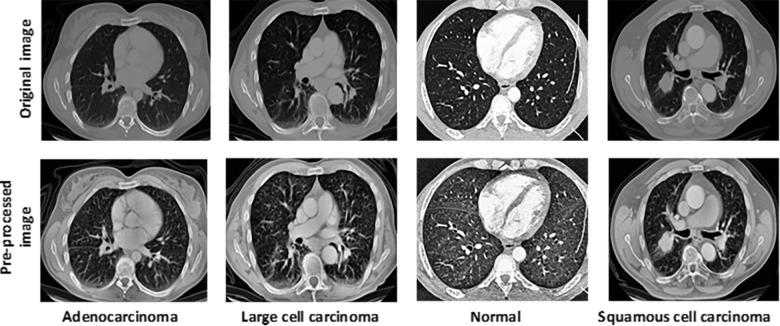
Lung cancer CT images before and after pre-processing.
